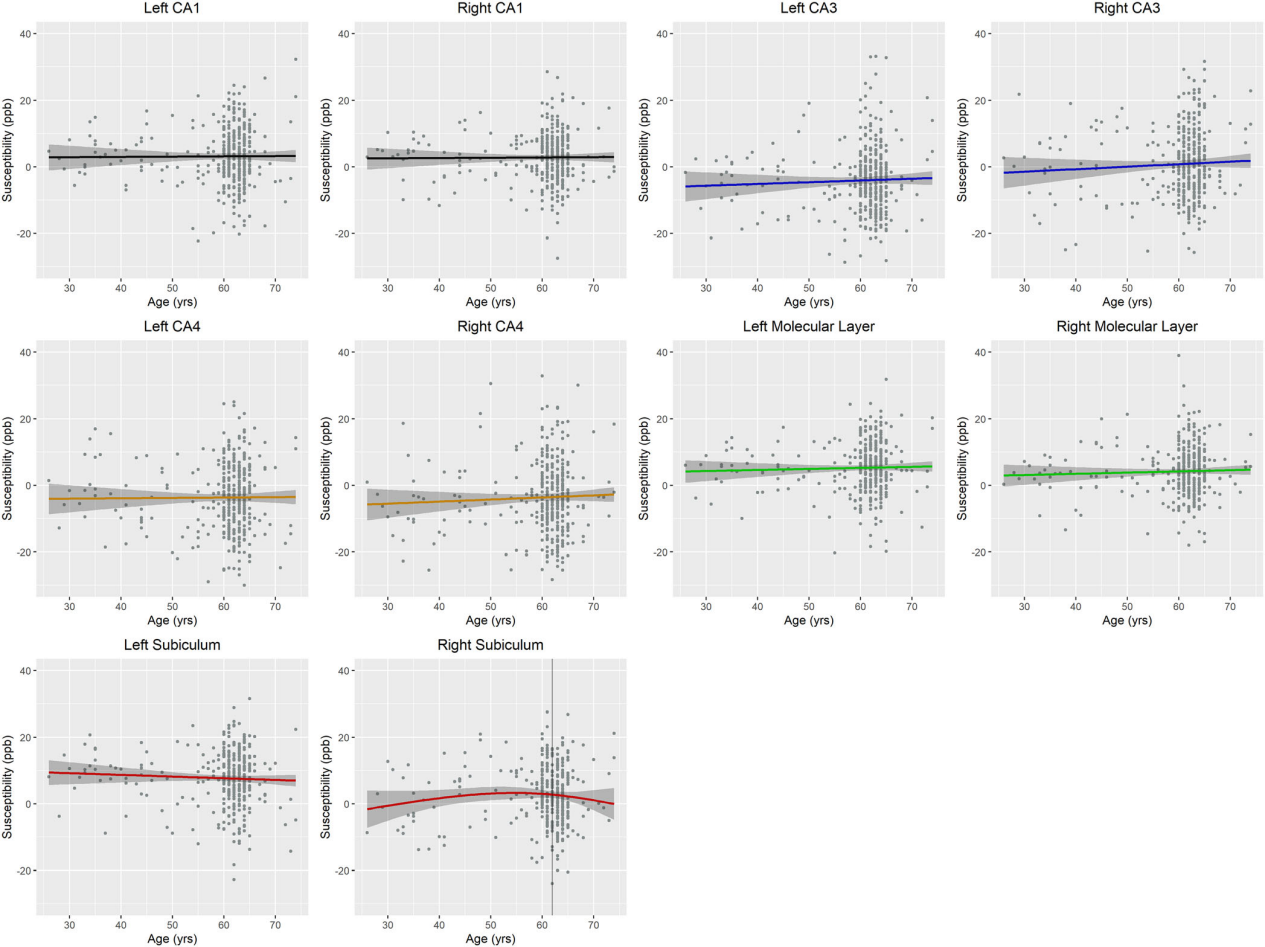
Relationships between iron content in hippocampal subfields as determined by quantitative susceptibility mapping on MRI and age at time of scan. None of these interactions reached statistical significance.
